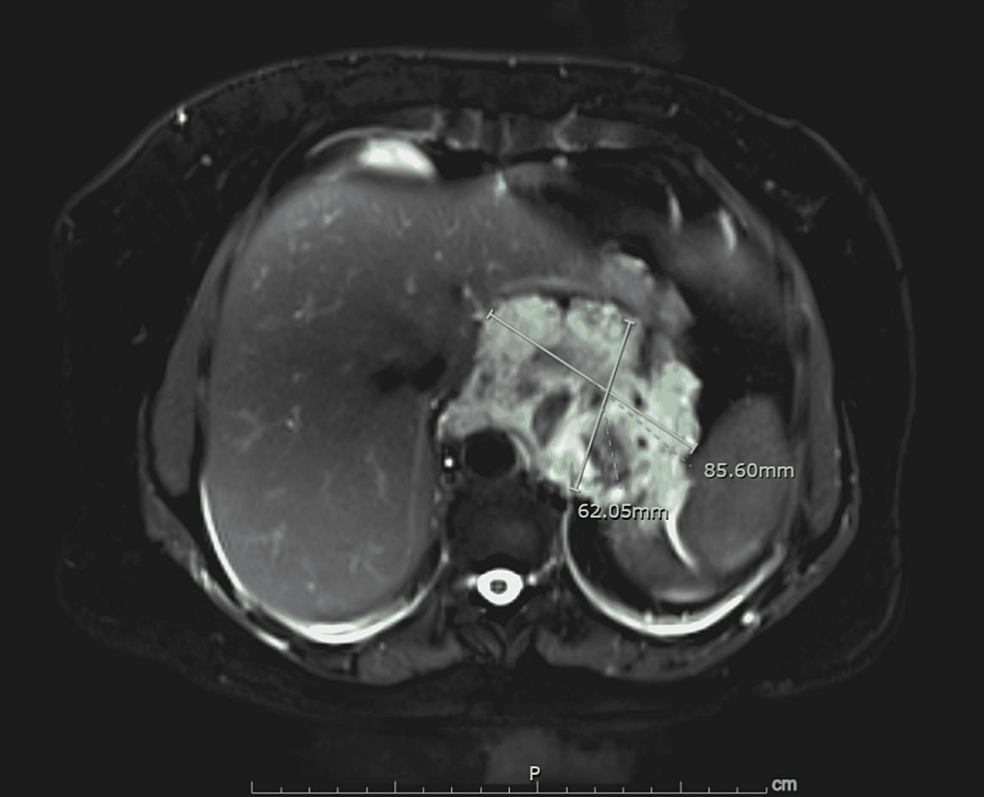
MRI abdomen showing low flow distal esophageal hemangioma
